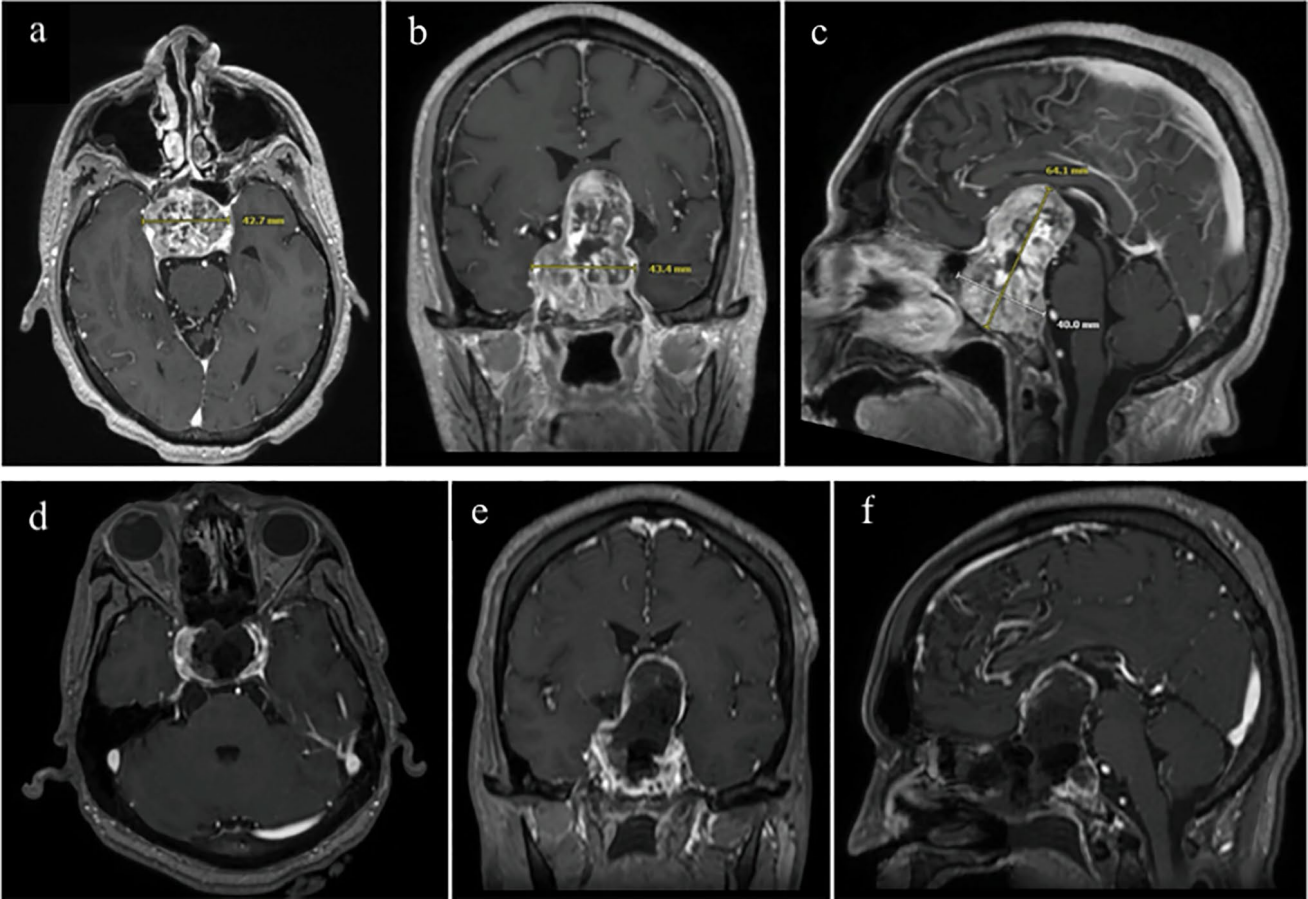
The images show radiological studies of the patient from the first case report. a, b & c Axial, Coronal, and Sagittal cuts from brain MRI (T1 C +) showing large sellar/suprasellar heterogenous mass, measuring 43.3 × 64.1×40mm, with diffuse contrast enhancement and signs of intralesional hemorrhages. It causes significant mass effects over the optic nerves/chiasm as well as invasion over the cavernous sinuses and clivus. d, e & f Axial, Coronal, and Sagittal cuts from intraoperative brain MRI (T1 C +) showing subtotal resection of large sellar/suprasellar mass, with little residual tumor on the right cavernous sinus
